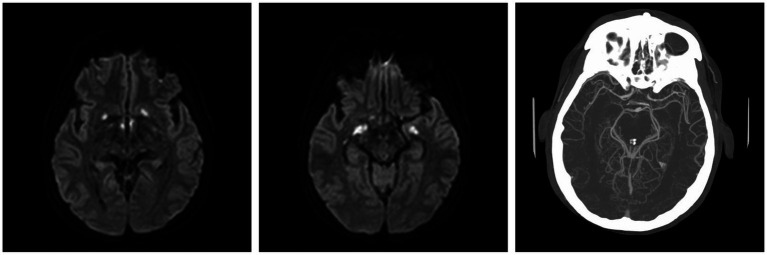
At the ED admission with altered mental status, MRI brain demonstrating basal ganglia and medial temporal infarcts. CTA head demonstrating multifocal stenoses involving bilateral ICA.
